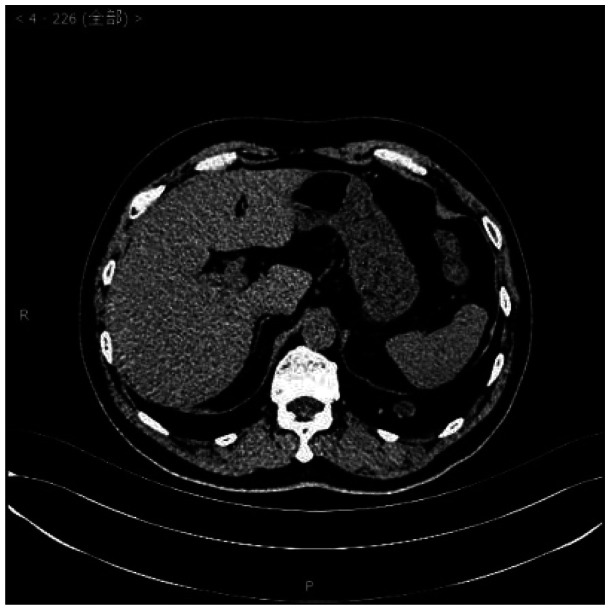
Chest computed tomography scan.
